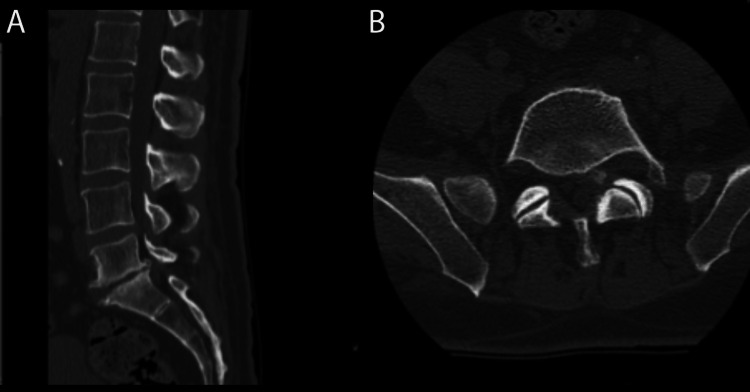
CT lumbar spine A. Sagittal view and B. Axial view exhibiting left paracentral partially calcified disc herniation
